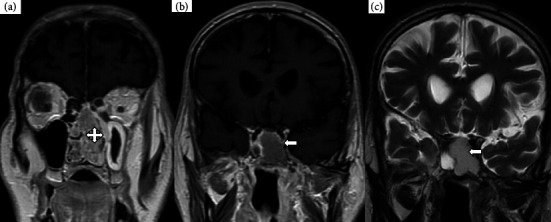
MRI facial bones coronal views: (a, b) postcontrast TI-weighted images and (c) T2-weighted images. The localization of the heterogeneously enhancing mass to the left nasal cavity can be seen (cross arrow). The sphenoid sinus contents (solid arrows) demonstrate a decreased signal intensity relative to the lateral ventricles on T2-weighted images while exhibiting an increased signal intensity on T1-weighted images. These findings align with the presence of retained secretions resulting from the obstruction of the drainage pathway caused by the mass within the left nasal cavity.
